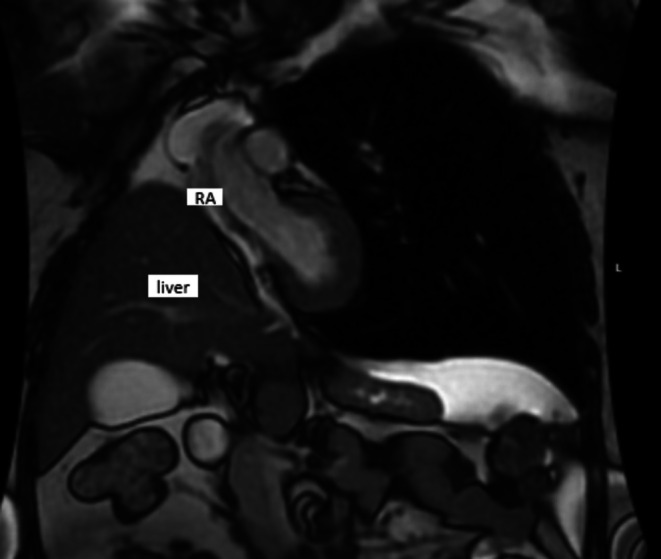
Cardiac MRI in the supine position showing remarkable right atrium collapse due to upward shift of the liver inside of the thoracic cavity.
